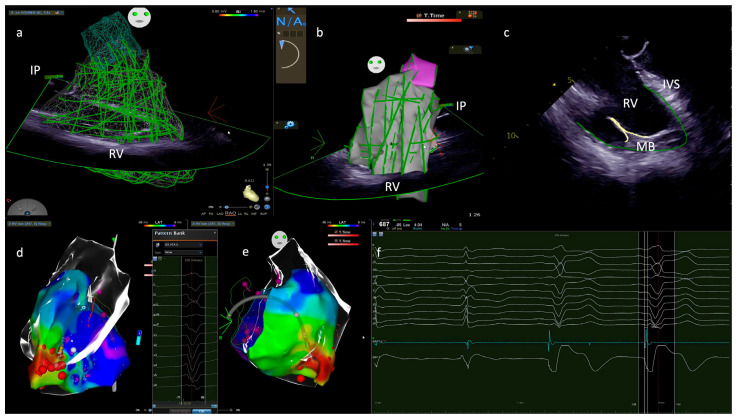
(a) Intracardiac echocardiography view; IP is in the right atrium in the right anterior oblique view; green lines paint the long and short axis of RV, constructing a 3D RV echo–map. (b) same image in the left anterior oblique view; (c) two−dimensional view of RV. The green lines trace the endocardial surface, and yellow lines the MB; (d) Merge of the 3D echo map and activation map of PVC in lateral view; (e) PVC activation map in right anterior oblique view; ablation at the earliest activation site on the MB using an electroanatomic mapping system; the red color on the activation map is the earliest activation site; the red bullets are the RF lesion tagged points; (f) electrogram PVC potential; sharp and QS unipolar signal and adequate PVC anticipation. IP: intracardiac probe; RV: right ventricle; MB: moderator band; 3D: three dimensional; PVC: premature ventricular complex; RF: radiofrequency.
